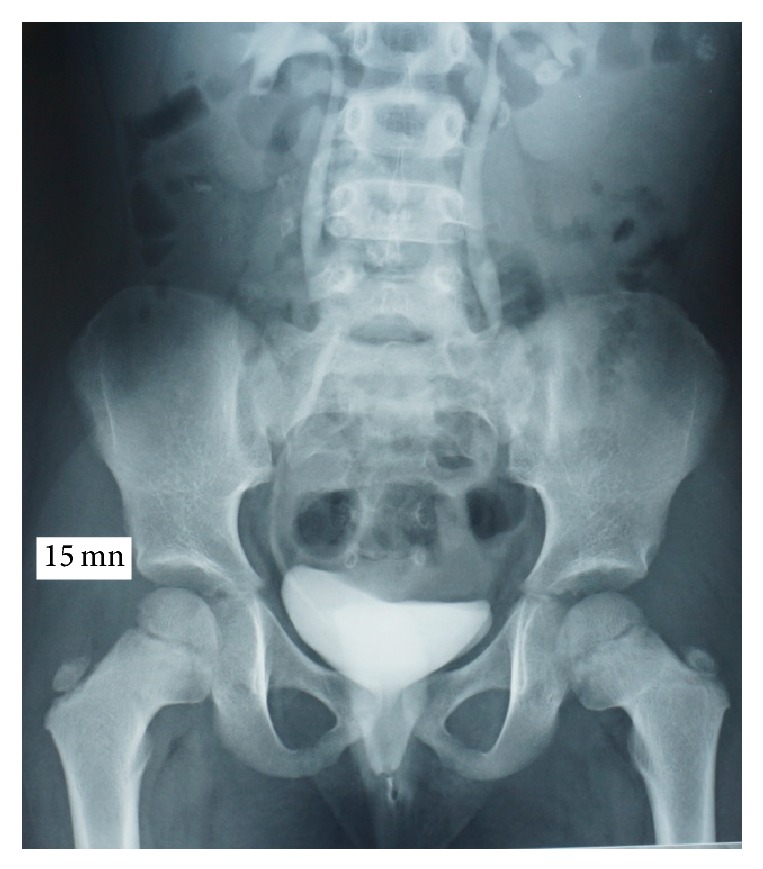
15 min IVU radiography. Bladder filling and vagina opacification.
